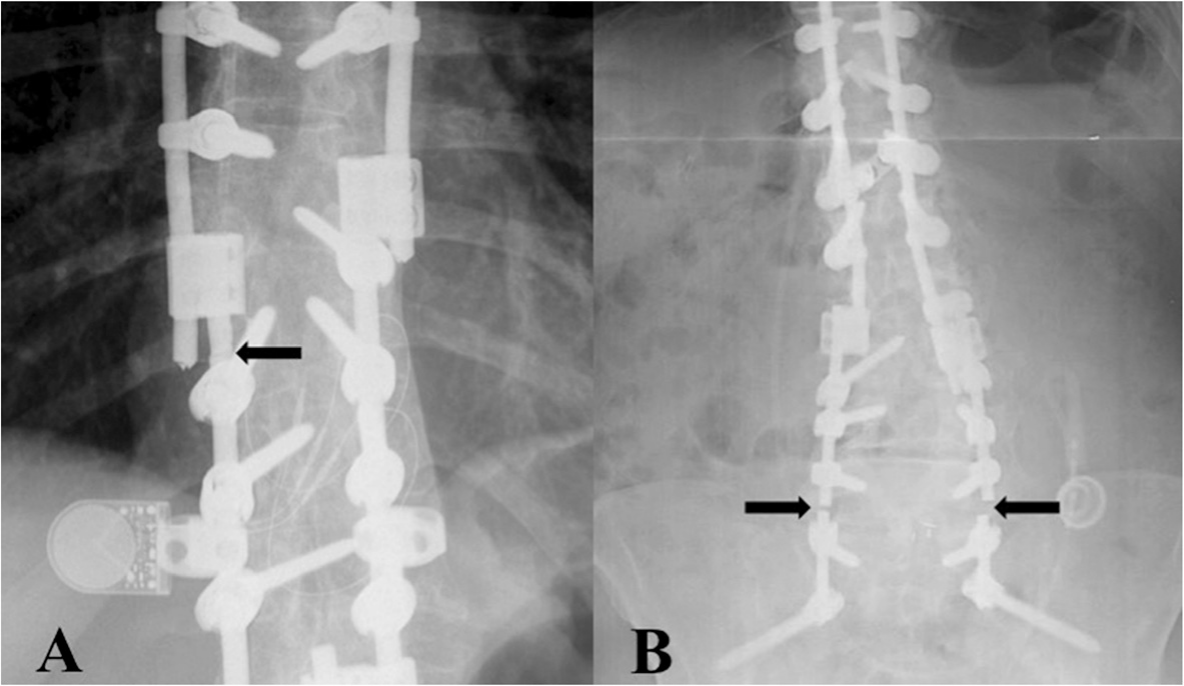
Radiographic findings of rod fracture. a Right T10 rod fracture near domino connector (rod fracture #6) diagnosed 58 months postop with associated T7-8 pseudarthrosis. This fracture occurred at location of prior cross-link. This patient had two subsequent PSO operations after the original PSO operation. The rod fracture occurred in a rod from the first PSO operation. Dominos were added during a subsequent PSO operation to connect to original instrumentation. b Bilateral L5-S1 rod fractures (rod fractures #1 and #2) diagnosed at 12 and 20 months post-operation with associated L5-S1 pseudarthrosis. These rods fractured at the apex of the rod bend with an 81° sagittal rod contour. Pseudarthrosis at L5-S1 was diagnosed during the second rod fracture at 20 months post-operation
